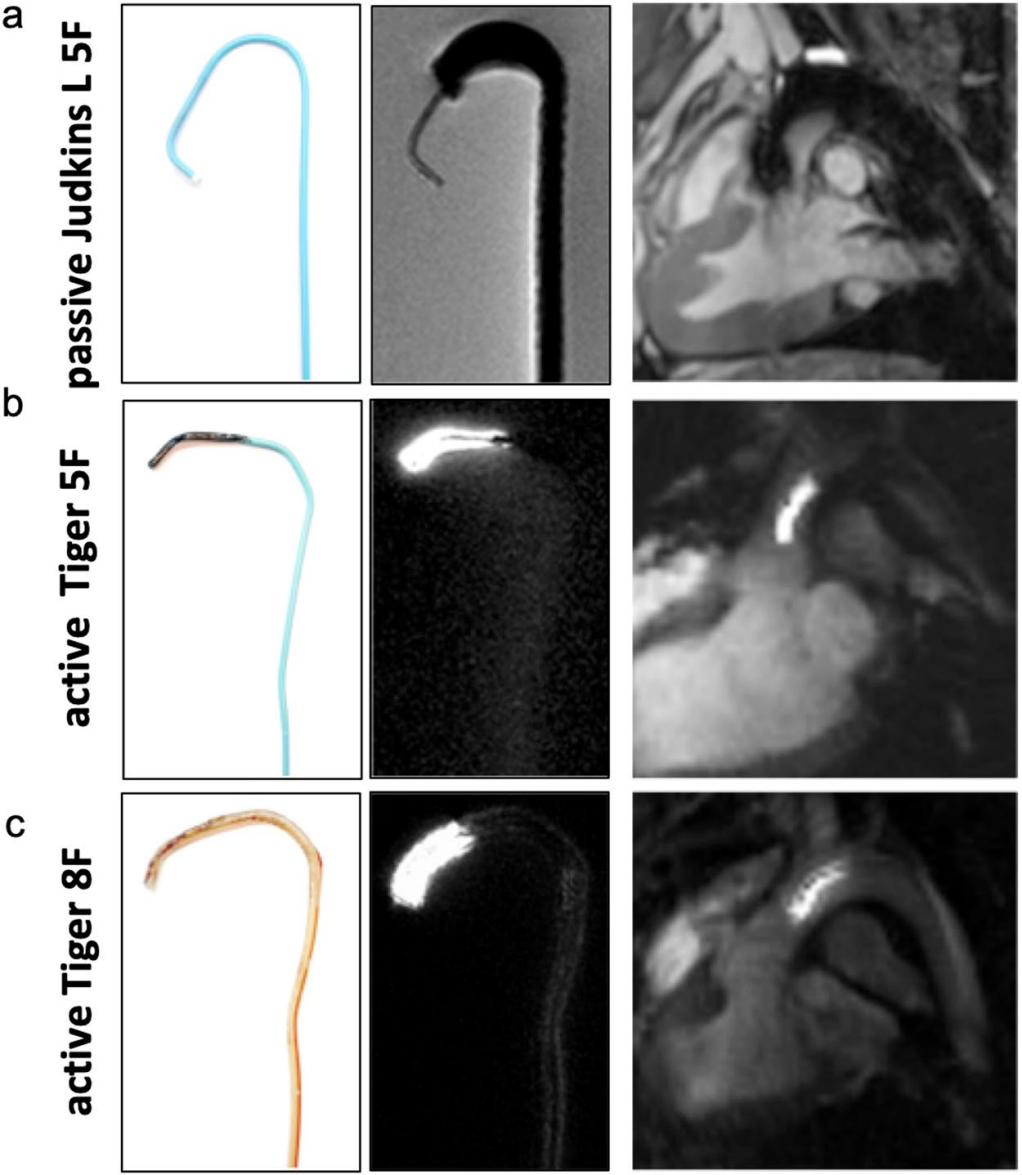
Comparison of guiding catheters for interventional MRI. Left Picture of the guiding catheter’s tip. Middle
